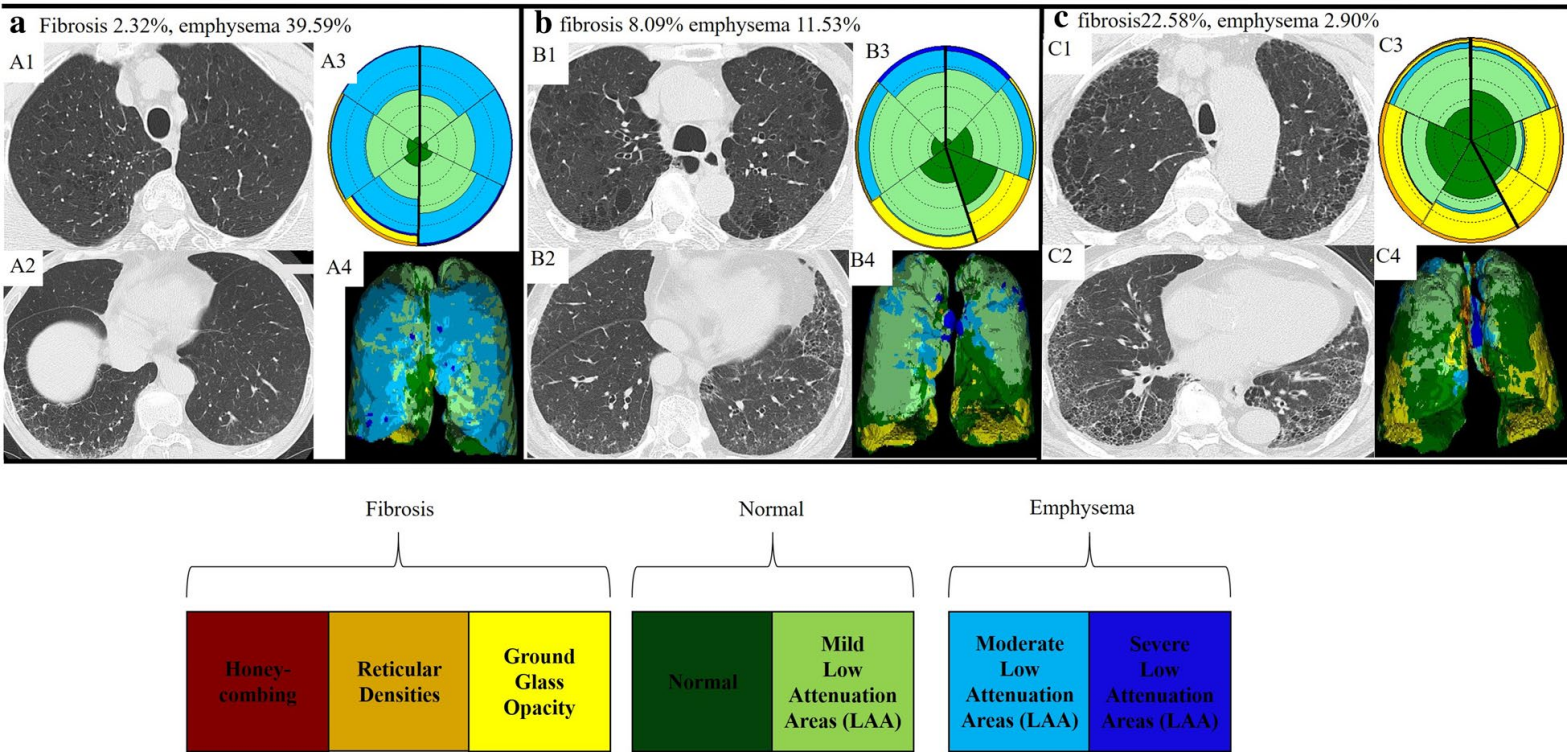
Representative high-resolution computed tomography images from three patients. Images of the upper lung (a1, b1, and c1) and lower lung (a2, b2, and c2) and 3D renderings analysed by computer-aided lung informatics for lung informatics pathology evaluation and rating shown on each right side (a3, 4; b3, 4; and c3, 4)
